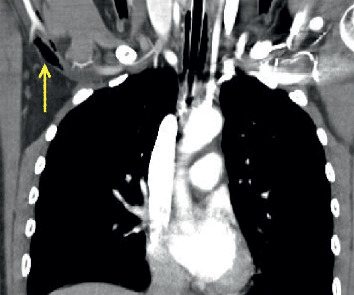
Coronal view of CT chest demonstrating air collection in the right axillary vein (arrow).
